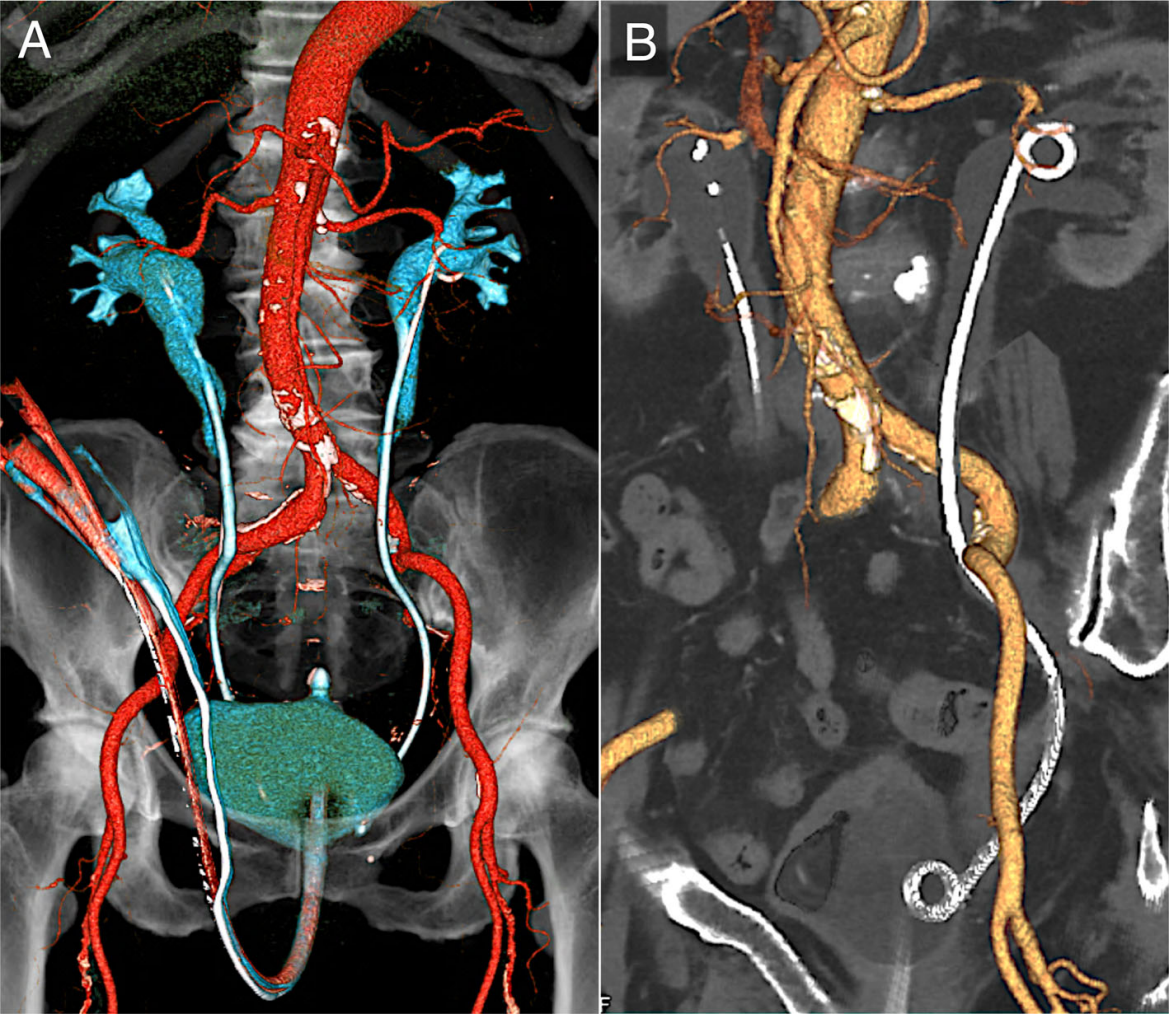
Computed tomography after the hemostasis of hematuria. a Computed tomography (CT) showed the crossing of the left ureter with a stent over the distal common iliac and internal iliac arteries. b CT imaging in the angulated 30-degree left anterior oblique position
